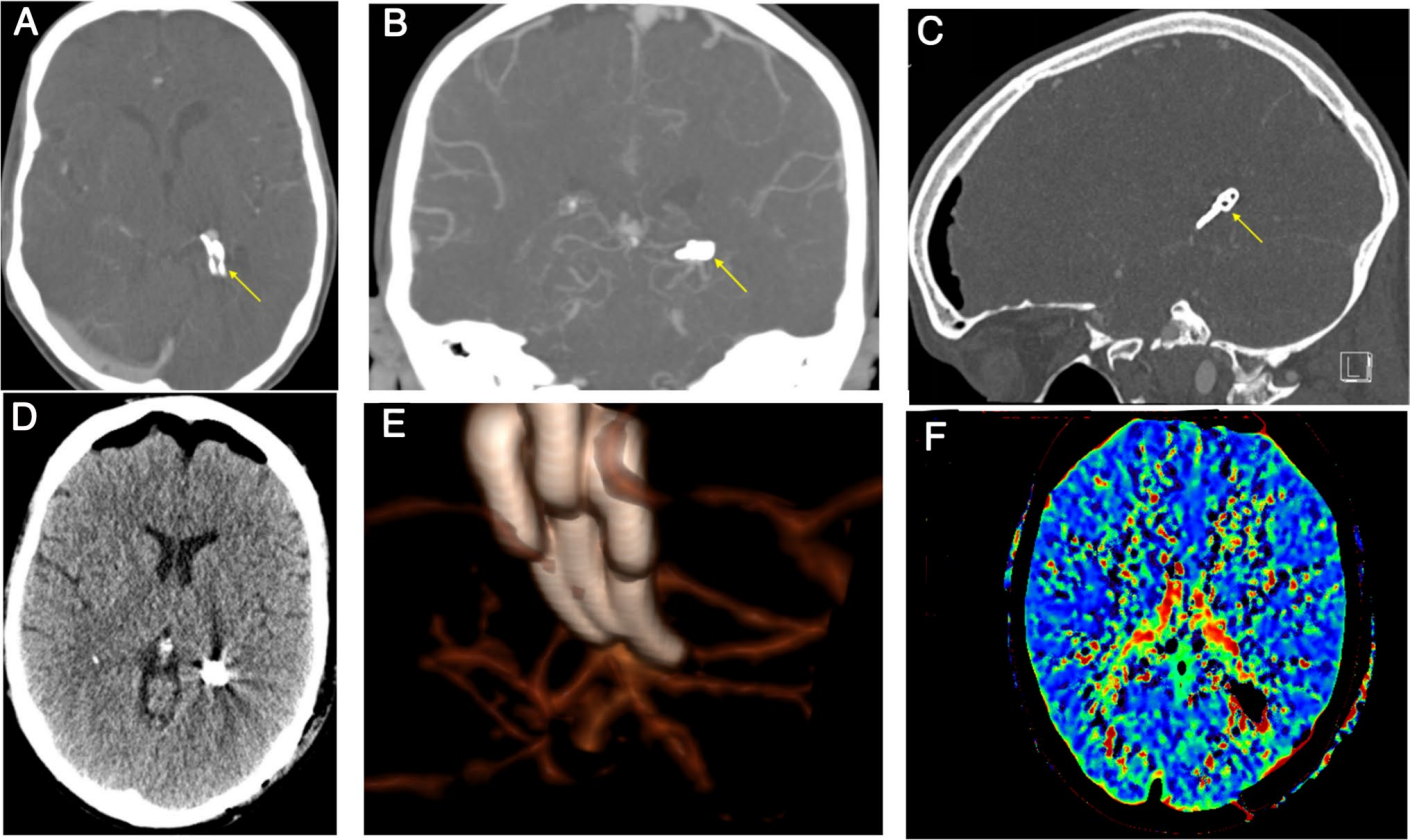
Postoperative images of case 1. A–C Axial, coronal, and sagittal CTA images show the position of aneurysm clips. D The postoperative CT scan show some postoperative intracranial air without any other complications. E Three clips were used to exclude the aneurysm and keep the patency of the vessels. F Postoperative perfusion CT scan shows that the area supplied by the PCA and its branches had a satisfactory outcome
